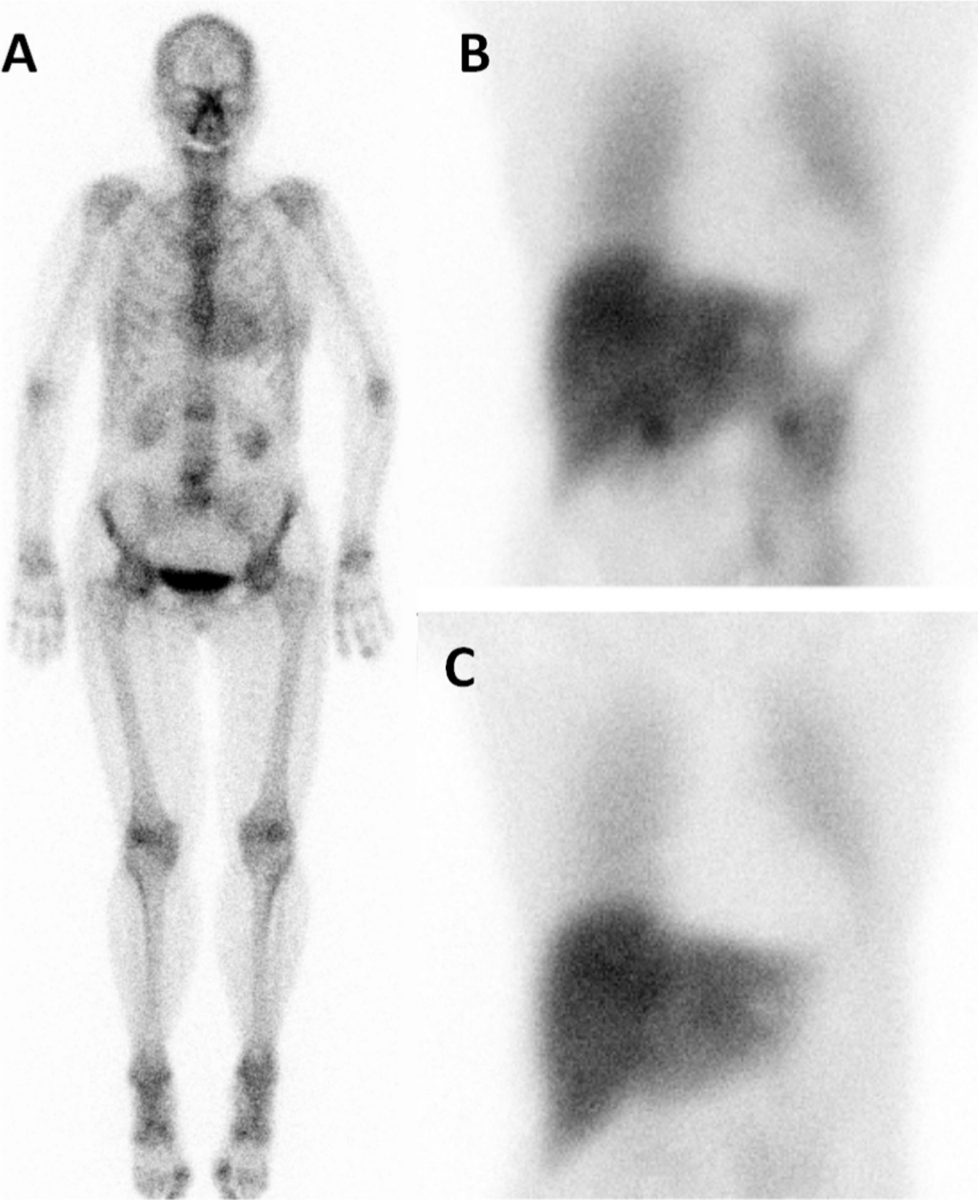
Example of a 70 year old female patient with ATTR amyloidosis based on Val30Met mutation, with both positive bone scan (A) and [I-123]-MIBG scintigraphy. B 15 minutes post injection (p.i.), C 4 hours p.i.. Late HMR 1.38, normal value in our laboratory: 2.0, performed with a medium energy collimator
